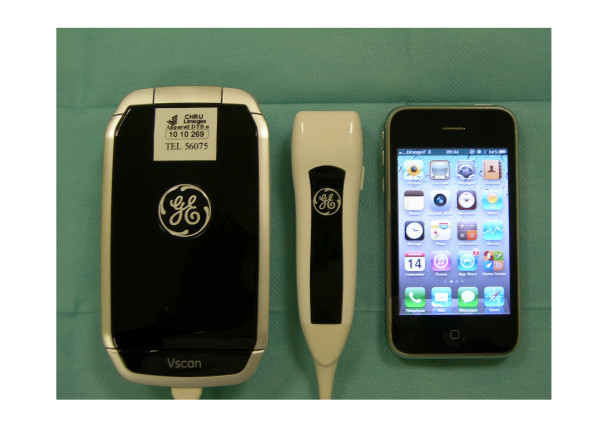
New generation miniaturized ultrasound system the size of a smartphone used in the current study.
